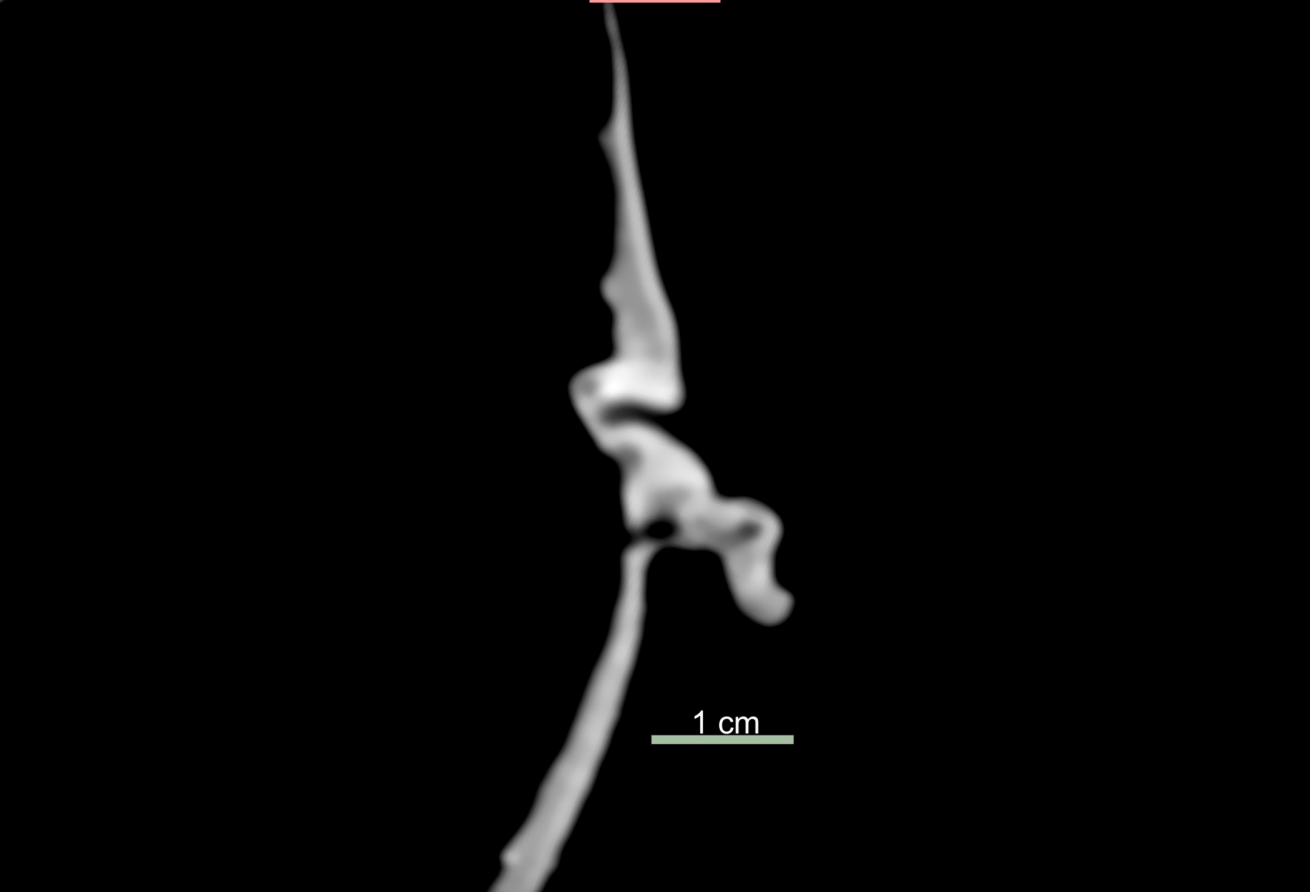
Comparative slice images of the right ear at different resolutions. Clinical CT axial slice of the right auditory canal and pinna.
